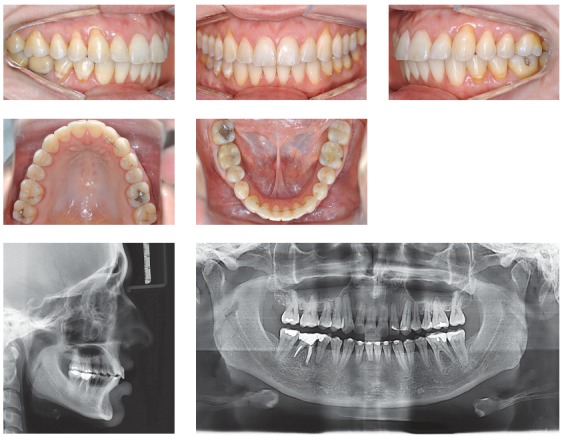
Final records: intraoral photographs, lateral cephalogram and panoramic radiograph.
